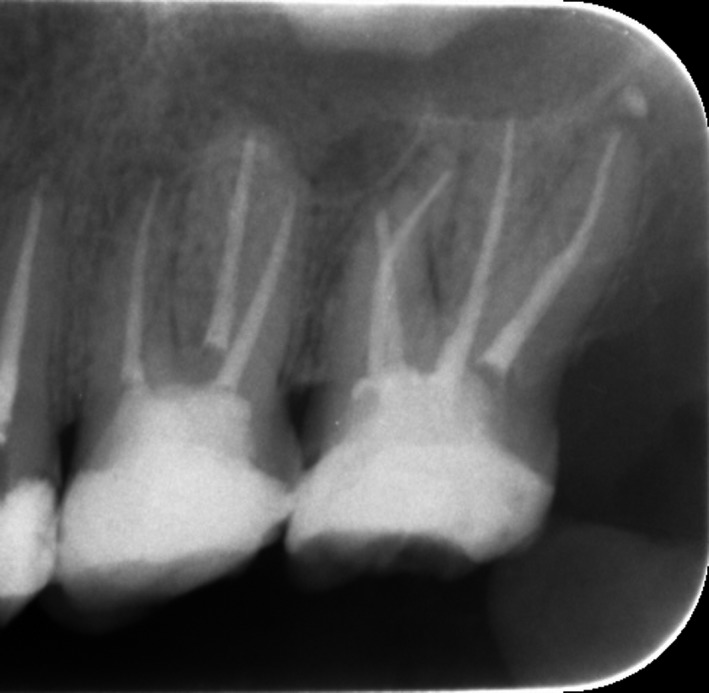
Postoperative Radiograph Showed Obturation of Both Teeth
